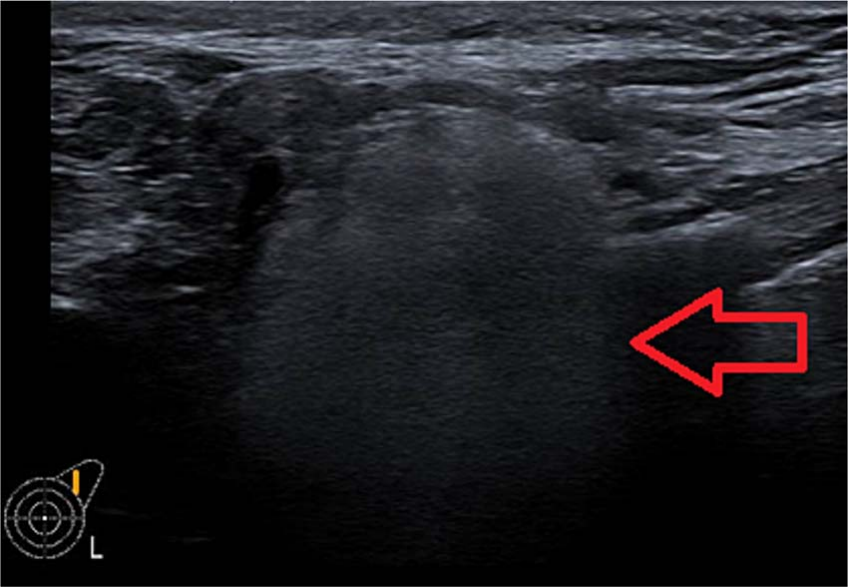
Ultrasound of the left axilla showed the typical appearance of the lymph node siliconoma.
